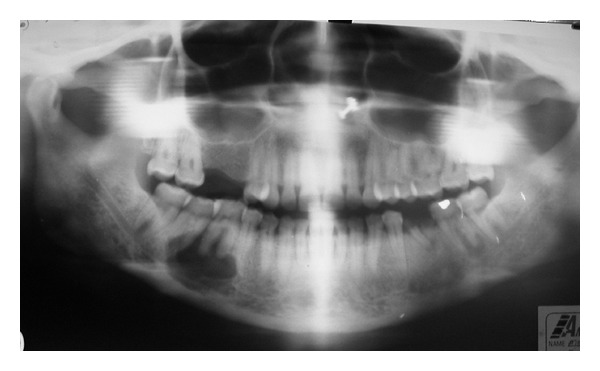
Orthopantomograph reveals a well-localized unilocular radiolucency in the mandibular first molar area on the right side involving the second premolar and second molar roots. Resorption of alveolar bone and loss of lamina dura around the first molar roots is also present.
